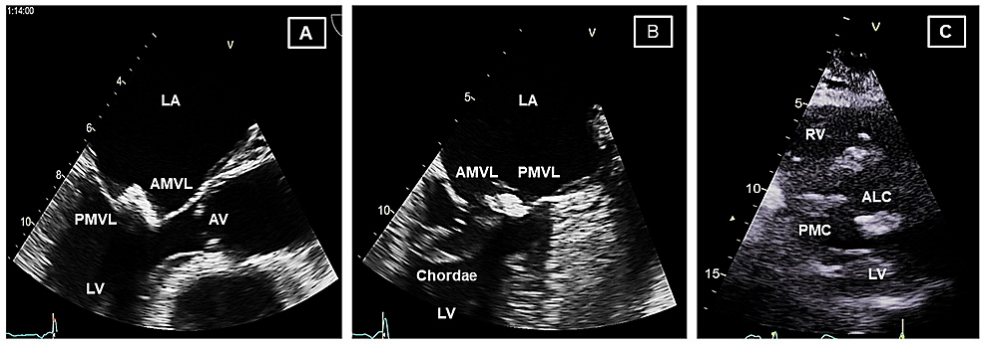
Representative images from the transesophageal (A, B) and transthoracic (C) echocardiographic studies showing severely calcific and stenotic mitral valve. (A) Mid-esophageal long axis view (120°); dense calcification involving the tips of the anterior and posterior mitral valve leaflets. Mitral valve motion is severely restricted. (B) Mid-esophageal two chamber view (90°); dense calcification is again noted to involve the tips of the anterior and posterior mitral leaflets and marked thickening of the chordal apparatus). (C) The parasternal short axis view at the level of the mitral valve showing marked calcification at the anterolateral and posteromedial commissures. ALC; anterolateral commissure, AMVL; anterior mitral valve leaflet, LA; left atrium, LV; left ventricle, PMC; posteromedial commissure, PMVL; posterior mitral valve leaflet. RV: right ventricle
